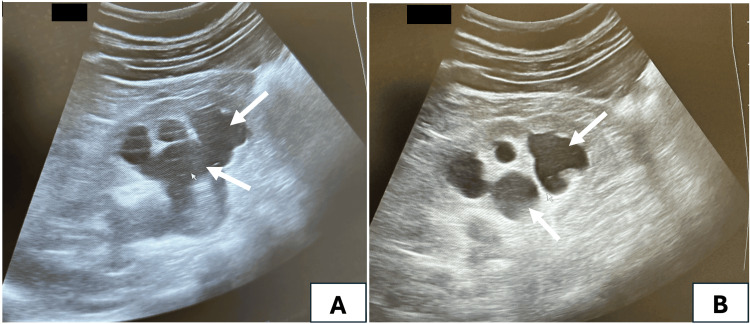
Renal ultrasound showing bilateral hydronephrosis (arrows) A: right kidney pyelocalyceal dilation; B: left kidney pyelocalyceal dilation
